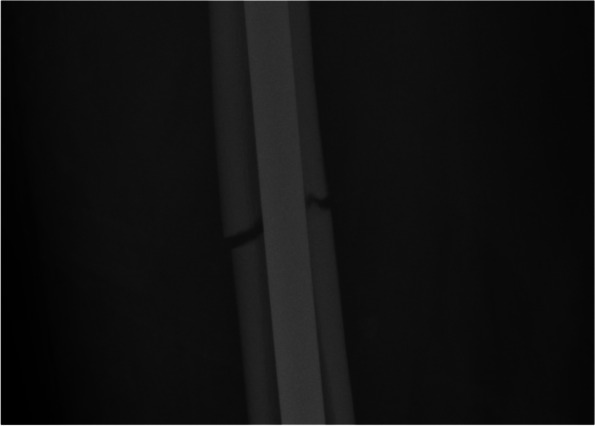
Radiographic example for CN difference 1 ~ 2 mm
